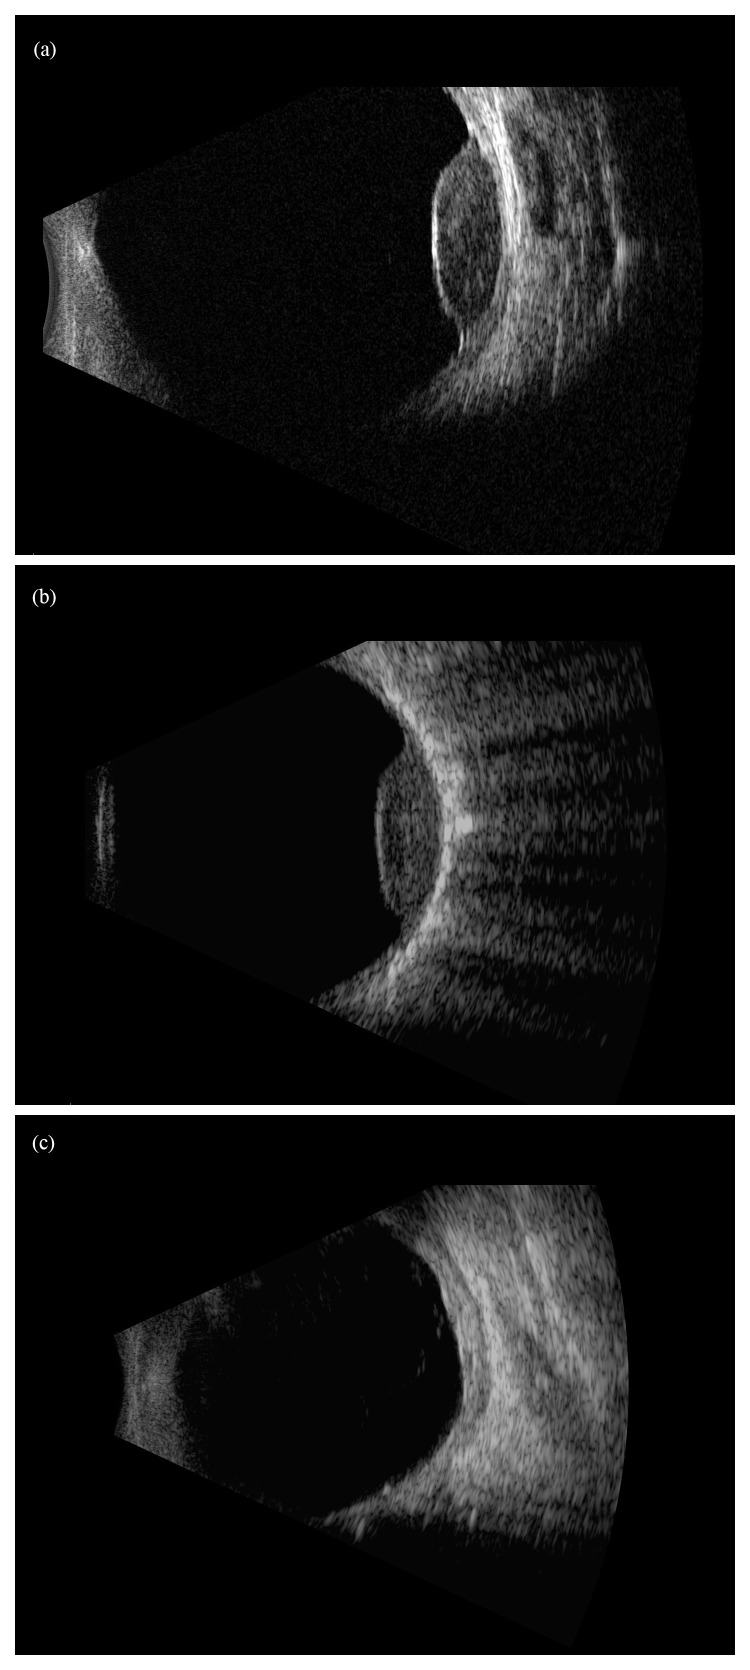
Uveal melanoma of a 66-year-old patient before and after plaque brachytherapy. (a) B-scan ultrasound image of the right eye before the plaque implantation. (b) B-scan ultrasound image of the same eye intra-operatively, demonstrating full coverage of the tumor with the plaque. (c) B-scan ultrasound image of the same eye 3 years after the plaque therapy, demonstrating regression of the tumor. This figure was reused from Schefler A and Kim R^48^ under the terms of CC BY 4.0 (https://creativecommons.org/licenses/by/4.0/).
